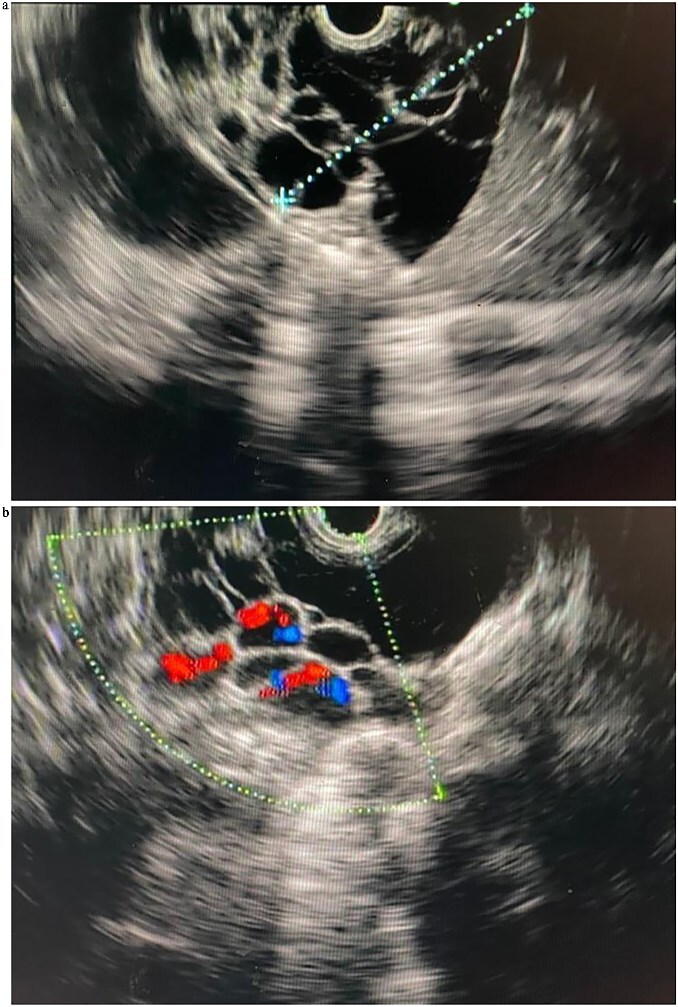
(a) Linear EUS (Olympus ME 1) views of the pancreatic lymphangioma, seen as cystic lesions in the pancreatic parenchyma. (b) Linear EUS (Doppler) (Olympus ME 1) views of the pancreatic lymphangioma, seen as cystic lesions in the pancreatic parenchyma.
